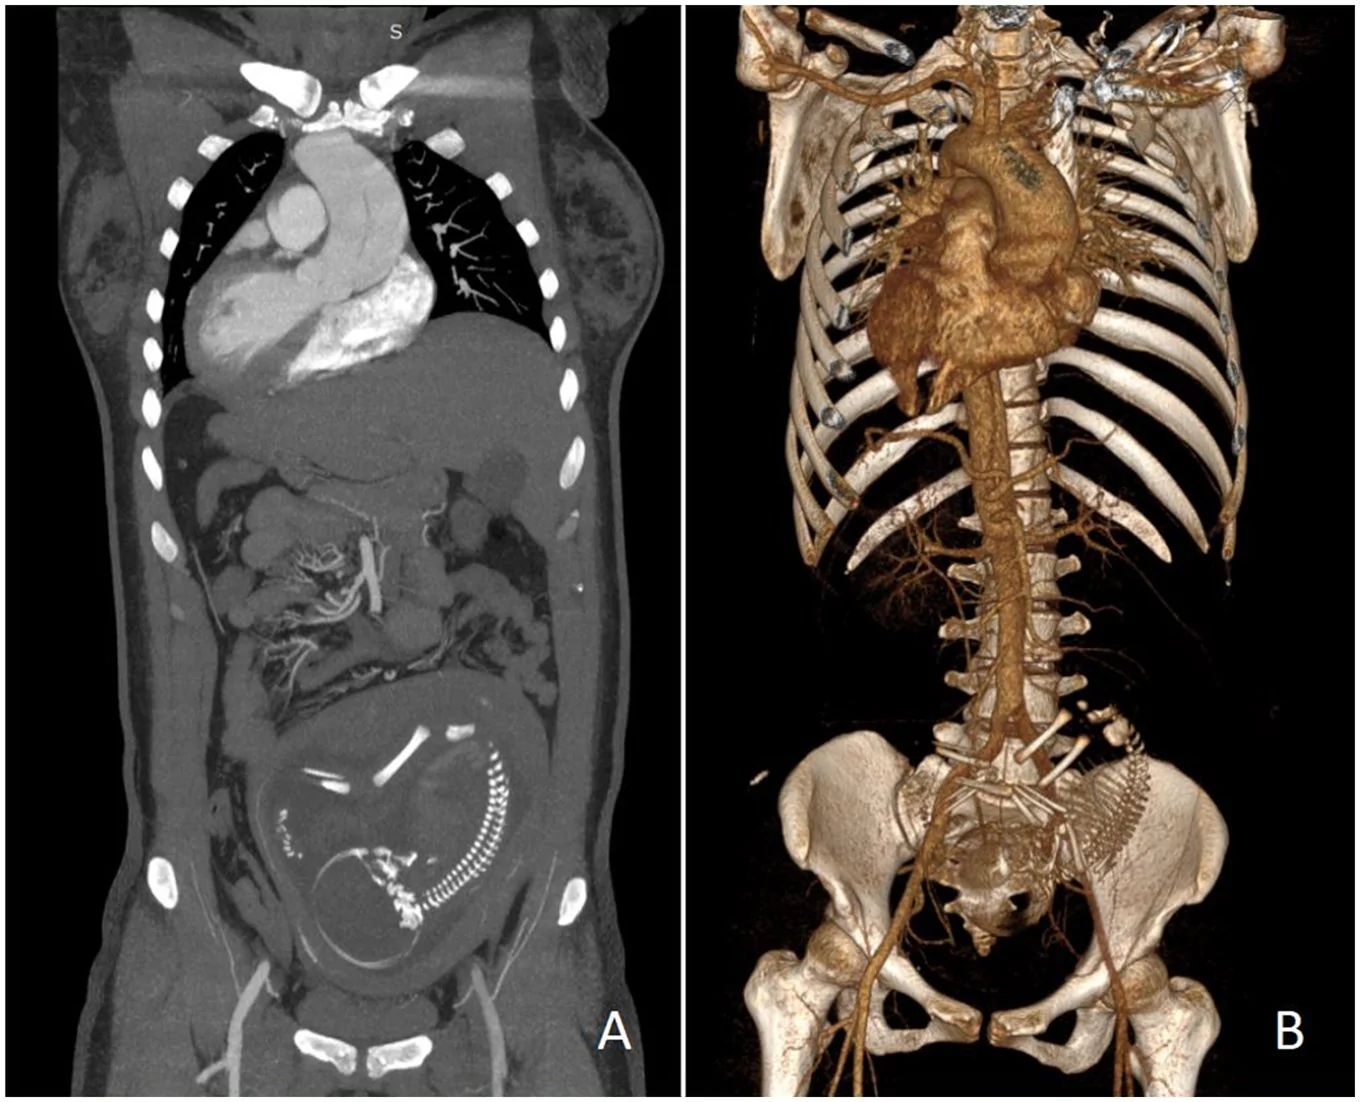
Preoperative Computed Tomography Angiography (CTA). (A) Coronal view showing situs inversus totalis with dextrocardia and a gravid uterus. (B) Three-dimensional reconstruction showing mirror-image anatomy and a reversed aortic arch.
